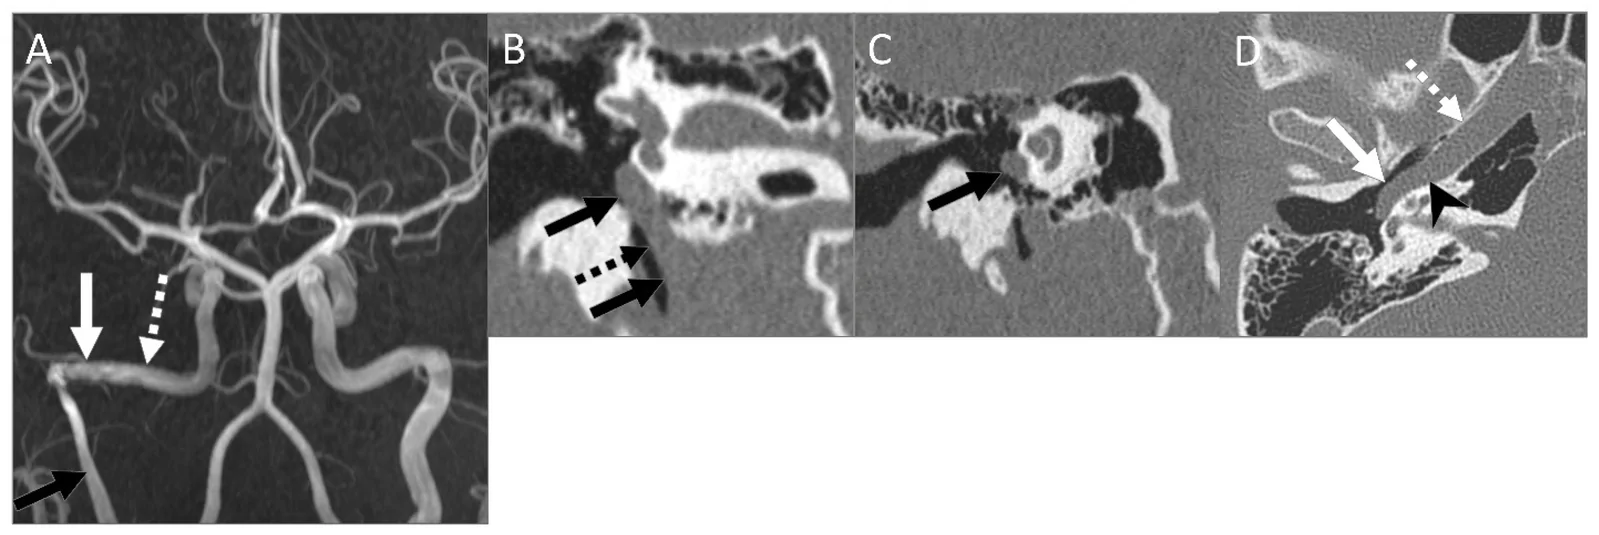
Aberrant right ICA. TOF MRA MIP (A) and coronal (B,C) and axial (D) high-resolution computed tomography (HRCT) images of the temporal bone show an enlarged inferior tympanic canaliculus (dashed black arrow). The inferior tympanic artery (black arrows) courses through the middle ear before joining the horizontal petrous ICA (dashed white arrows) via the caroticotympanic artery (white arrow). The aberrant collateral vessels demonstrate a lateralized course and a thin caliber with acute angulation, resembling a reversed number “7” on the coronal plane. The carotid plate is absent (black arrowhead) where the caroticotympanic artery (white arrow) connects to the normal horizontal portion of the petrous ICA (dashed white arrows).
